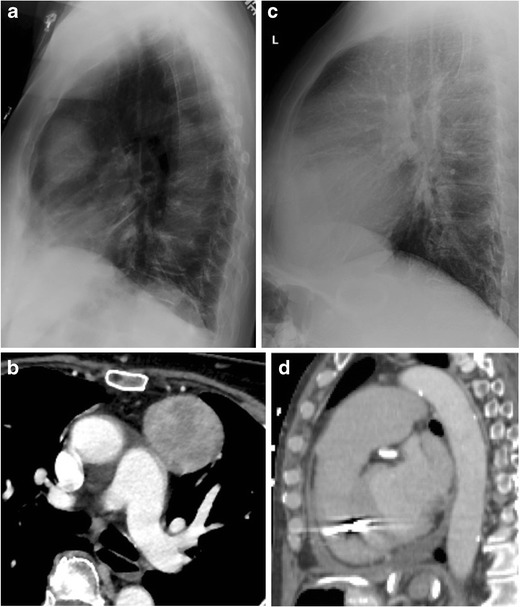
Two examples of classic retrosternal clear space pathology: a lateral chest radiograph (a) and axial CT (b) showing an anterior mediastinal mass, and a lateral chest radiograph (c) and sagittal CT (d) showing an enlarged right ventricle and main pulmonary artery in a patient with pulmonary hypertension
